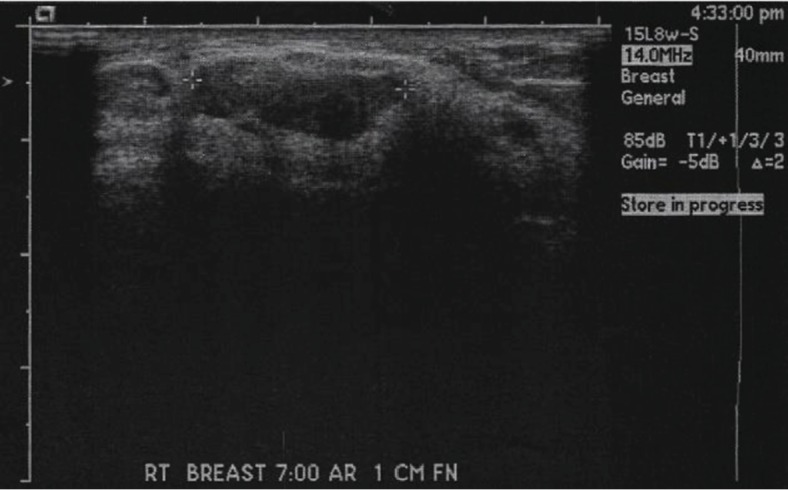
Ultrasound of the right breast before fine-needle biopsy shows a nodular density with multiple areas of hyperechoic densities within it.
